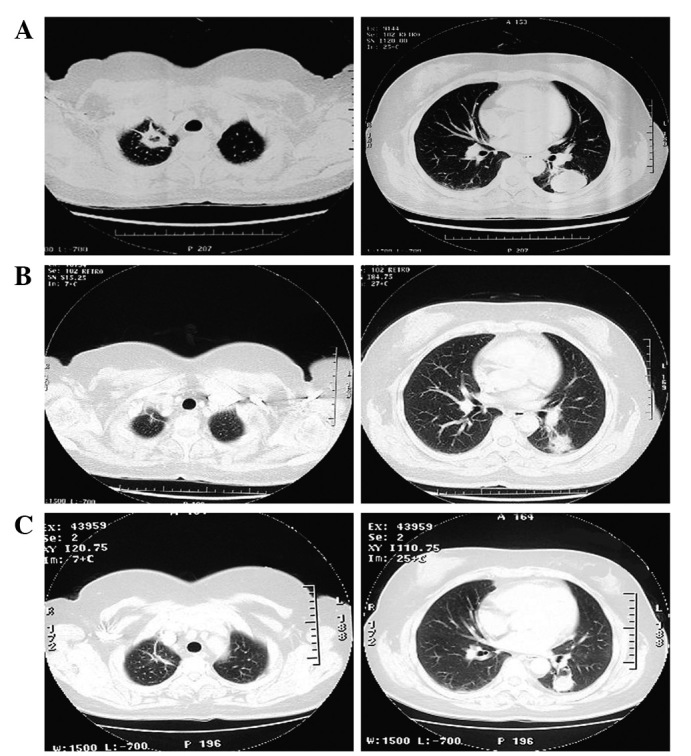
Computed tomography radiographs showing changes in pulmonary metastasis in response to erlotinib therapy. Axial and coronal views of a (A) baseline scan prior to starting erlotinib, (B) partial response after one month of erlotinib treatment and (C) stable disease after three months.
